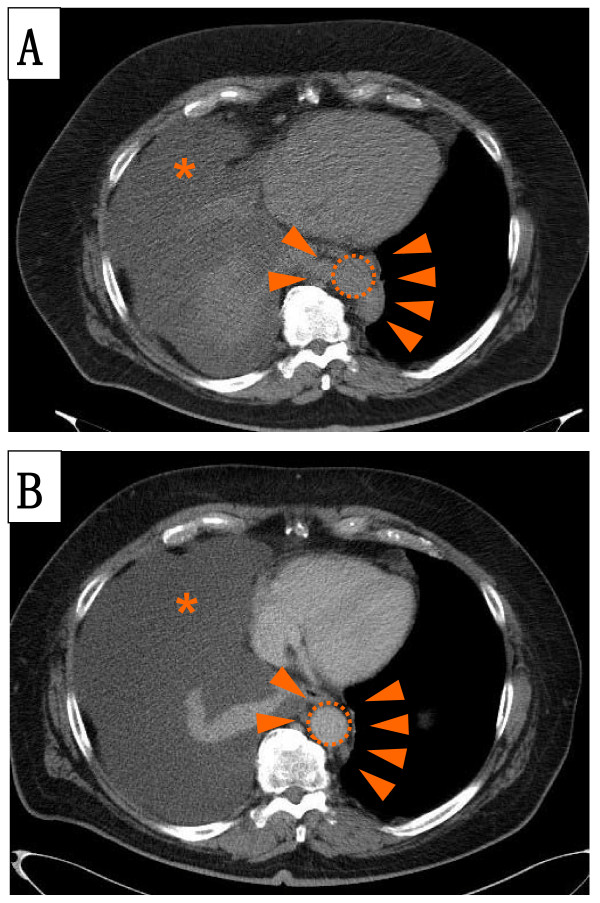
A CT scan demonstrates para-aortal lymphadenopathy (A) before and (B) after two cycles of immunochemotherapy. Para-aortal lymphadenopathy (arrow heads) might have been the most probable cause of the thoracic duct injury resulting in chylothorax (asterisk). Immunochemotherapy reduced the lymphadenopathy after only two cycles. Nevertheless, the pleural effusion (asterisk) still persisted, indicating that the thoracic duct injury had not healed. The aorta is indicated (dotted circle).
